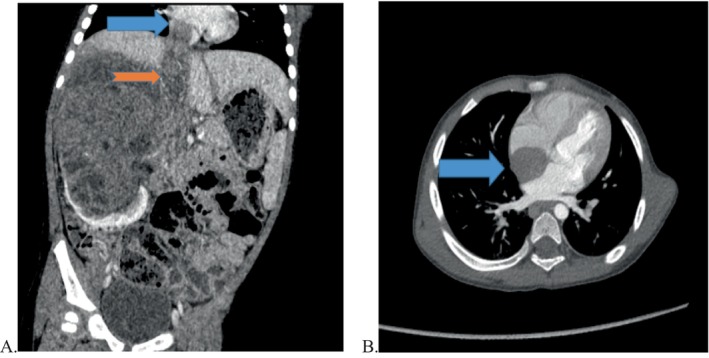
Preoperative computed tomography (CT) revealed a tumour thrombus in the inferior vena cava (IVC) (orange arrow in A) and a tumour thrombus in the right atrium (blue arrow in A and B).
